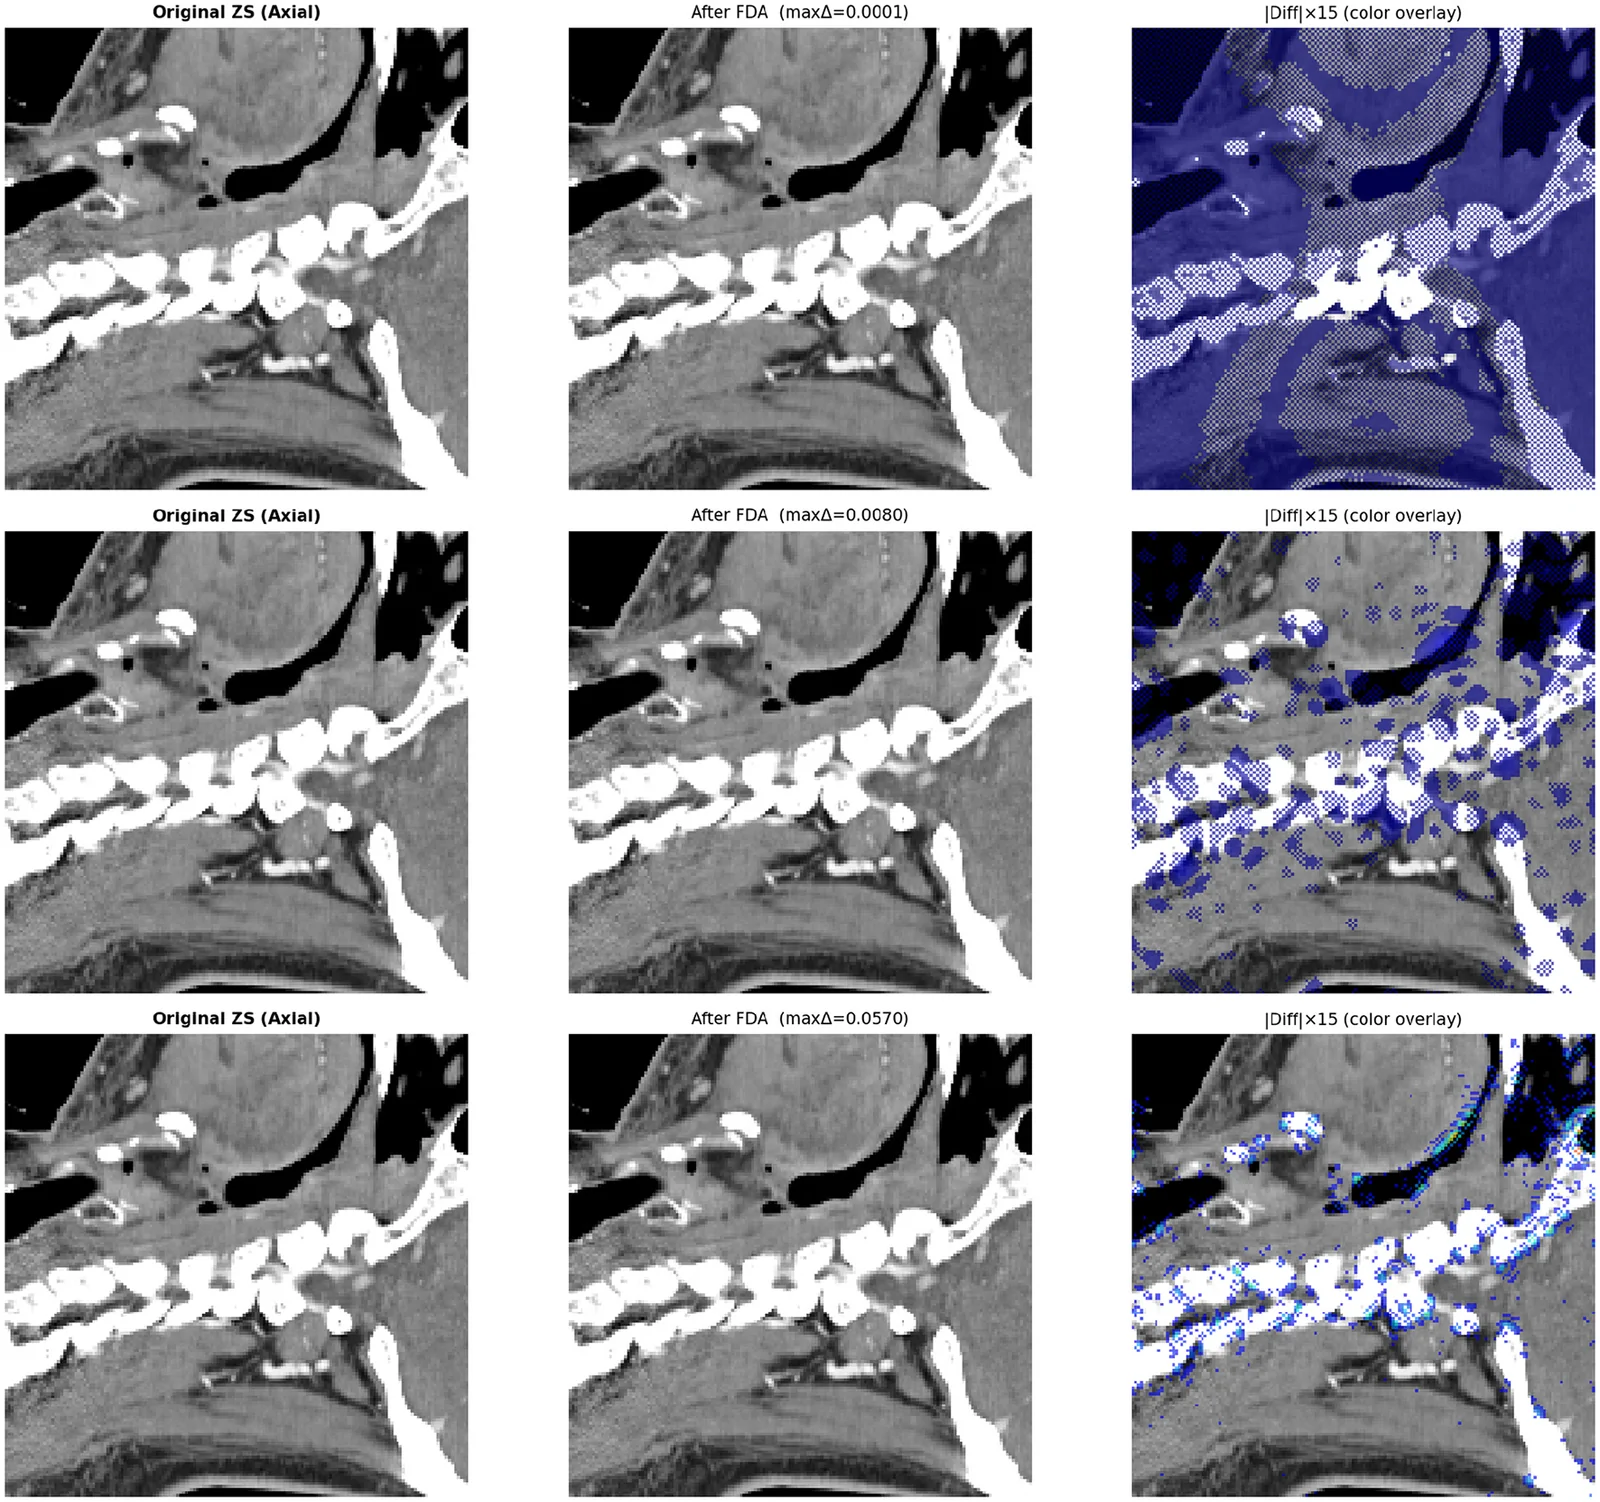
FDA style perturbation visualization on ZS axial CT slices. Left: Original slice; Middle: After FDA with maxΔ values; Right: Difference map × 15 (color overlay). Top to bottom: Increasing perturbation magnitudes—maxΔ = 0.0001 (negligible), maxΔ = 0.0080 (our optimal β ∈ [0.01, 0.02], preserving anatomy while altering global style), and maxΔ = 0.0570 (excessive, causing boundary blur). The selected range achieves style diversity without disrupting anatomical integrity.
